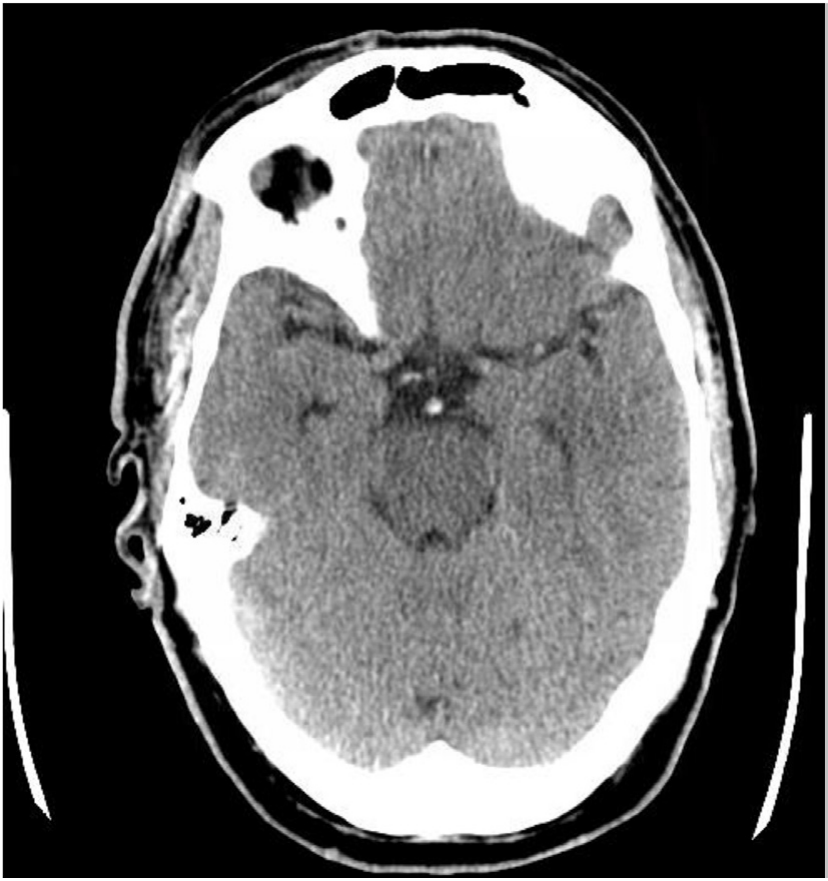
Hyperdense basilar artery (HDBA) sign. Axial non-enhanced computed tomography in a 56-year-old man who presented with unresponsiveness. Hyperdensity is visualized in the basilar artery consistent with a HDBA sign.
